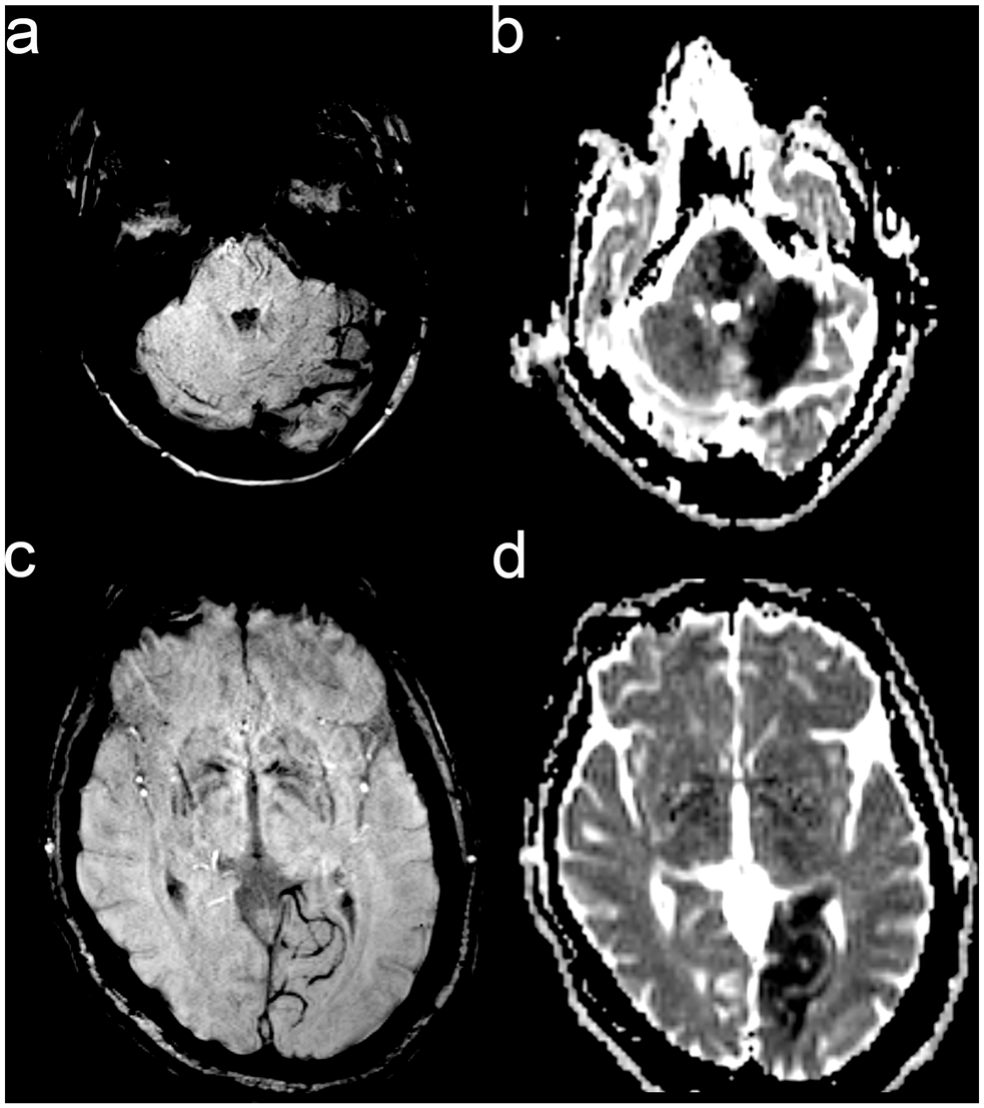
SWI changes in basilar artery occlusion. MRI was performed in two different patients after endovascular recanalization in basilar artery occlusion. Hypointense and enhanced vessels are displayed in the brain stem and cerebellum of one patient (a) and in the PCA-territory of another patient (c). Corresponding DWI changes appear on apparent diffusion coefficient maps in the same region (b, d).
